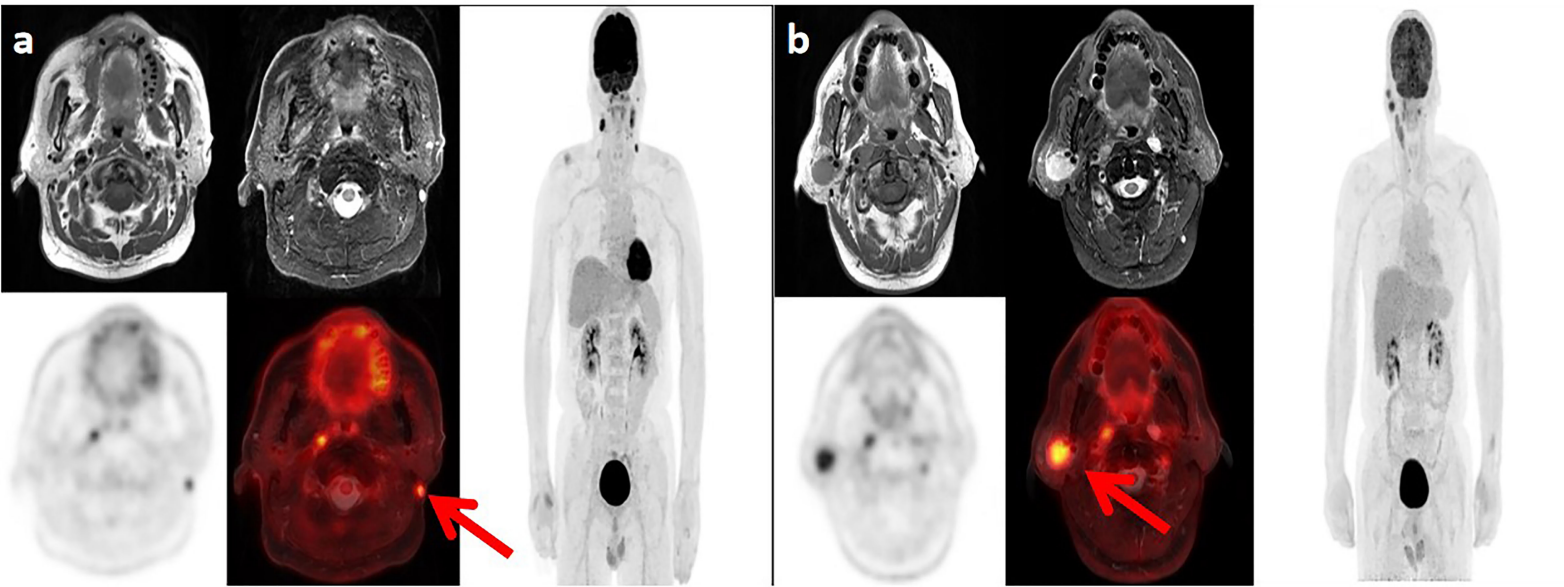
Lymph node metastases in two patients with nasopharyngeal carcinoma. (A) Positron emission tomography (PET)/magnetic resonance imaging (MRI) in a 62-year-old man show the left level Xa lymph node metastasis. (B) PET/MRI in a 47-year-old man show the right level VIII lymph node metastasis.
